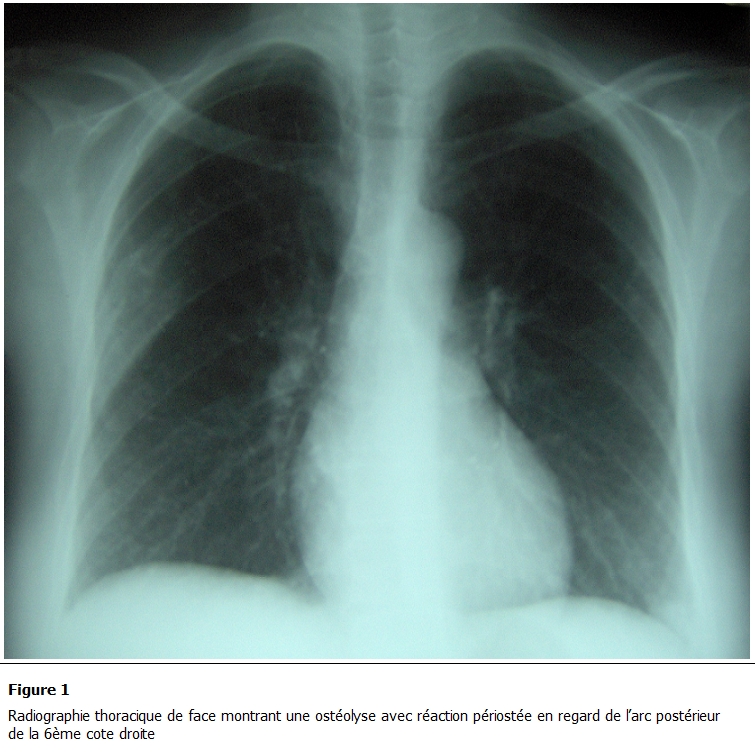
radiographie thoracique de face montrant une ostéolyse avec réaction périostée en regard de l’arc postérieur de la 6^ème^ cote droite.
